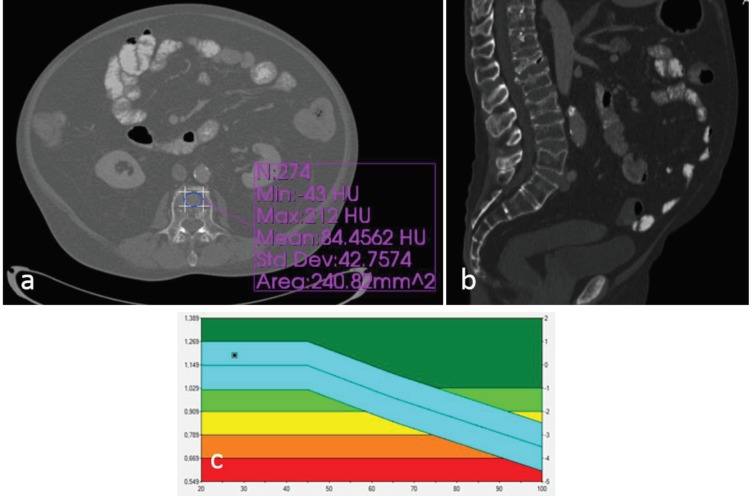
A patient with osteoporotic vertebrae fractures. a) The abdominal CT density measurement from L3 vertebra on axial plane
